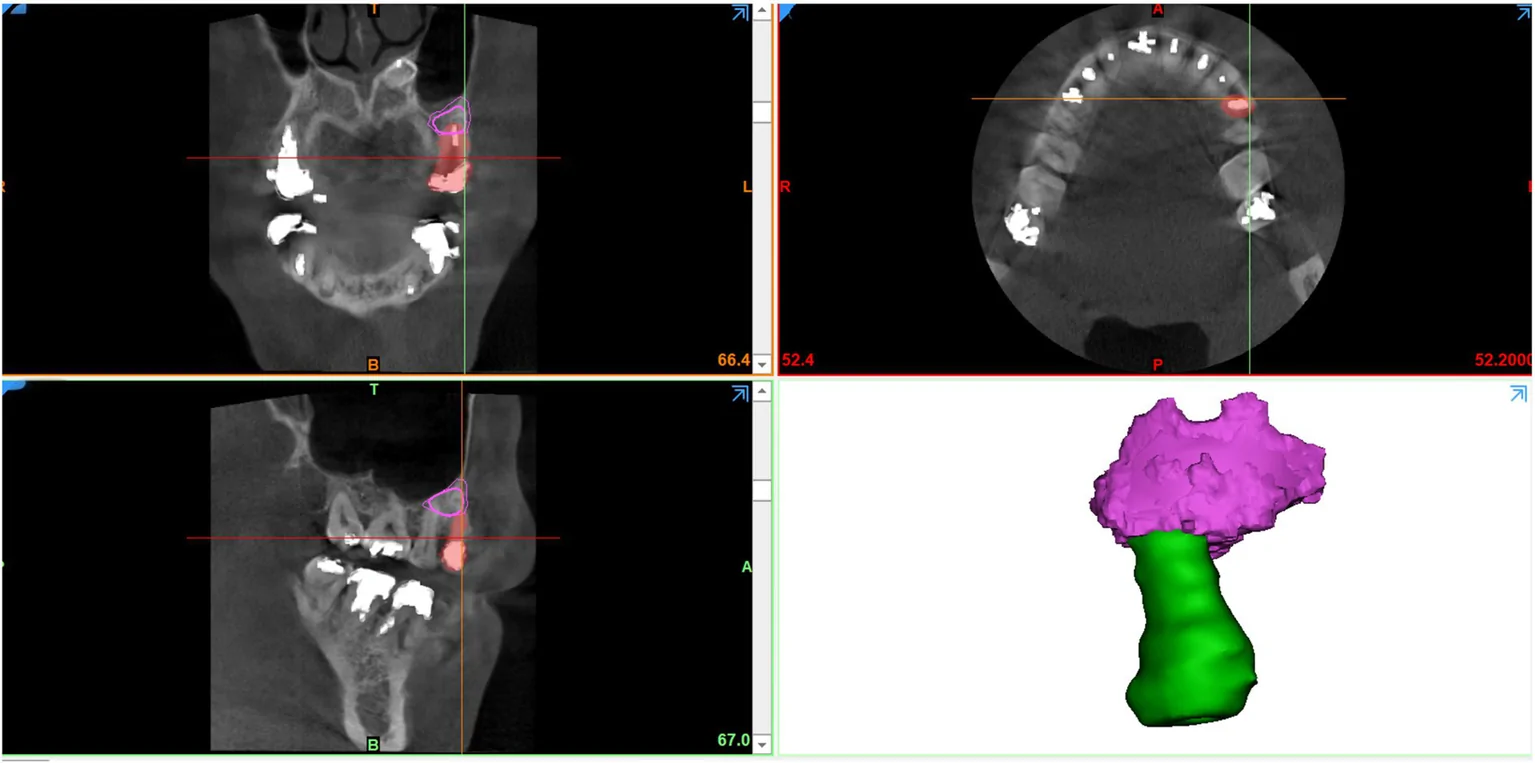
Complete healing of periapical lesion tissue with GBR; the hard tissue (purple) completely covered and encapsulated the resected apex (green) in 3D reconstruction by the Mimics.
